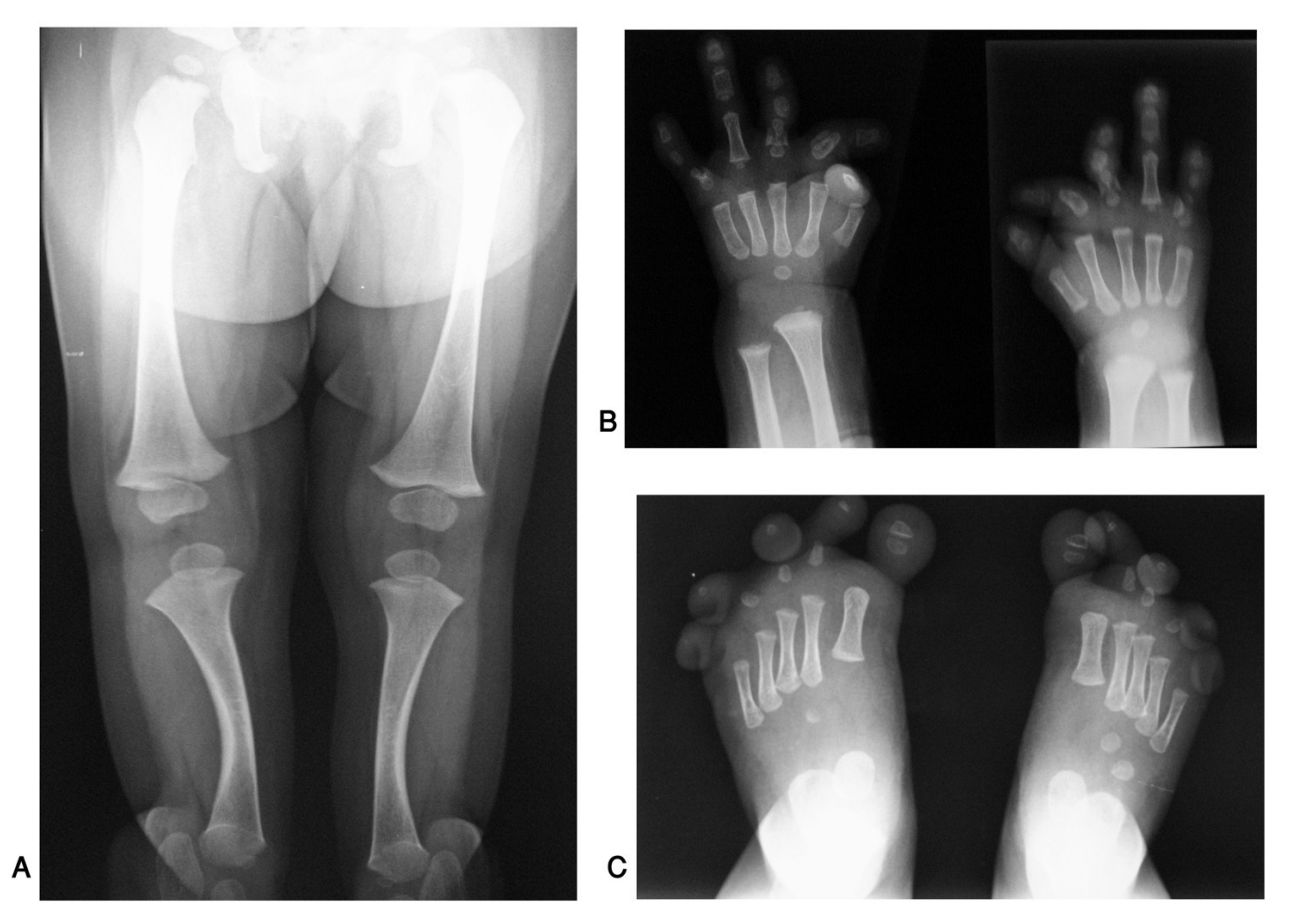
An Egyptian patient with du Pan syndrome. Note absence of both fibulae (A), X-ray of both hands and feet showing severe brachydactyly and radial deviation of fingers (B, C). (Limb Malformations & Skeletal Dysplasia Clinic, MSU, NRC).
